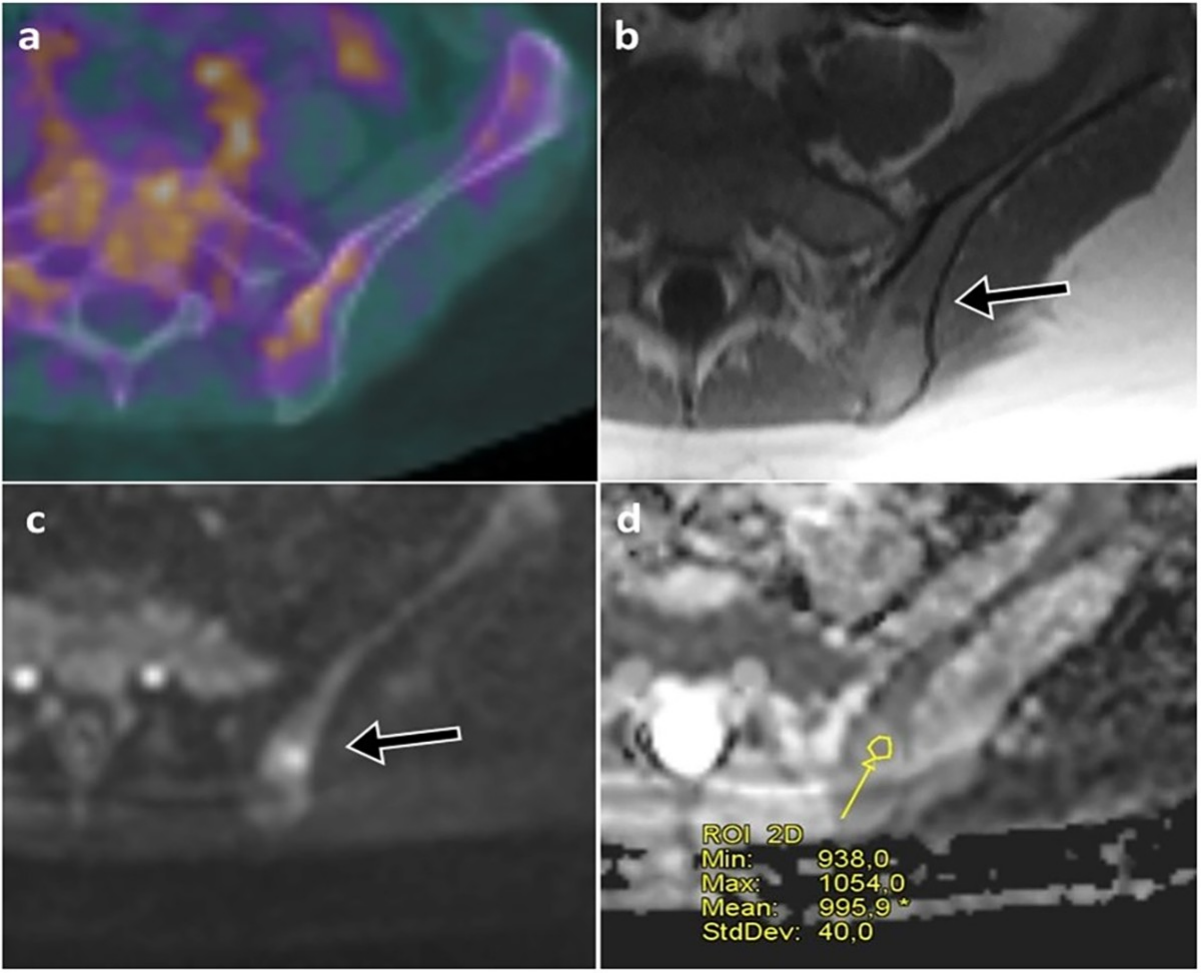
In the same pair of examinations of Fig 2, axial fused FDG-PET/CT showed non-specific FDG uptake in the pelvic bones (a). T1 weighted axial image from WB-MRI showed a suspicious bone lesion in the left iliac bone (arrow in b), which was hyperintense on b-900 DWI images (arrow in c) with low ADC values (d).
